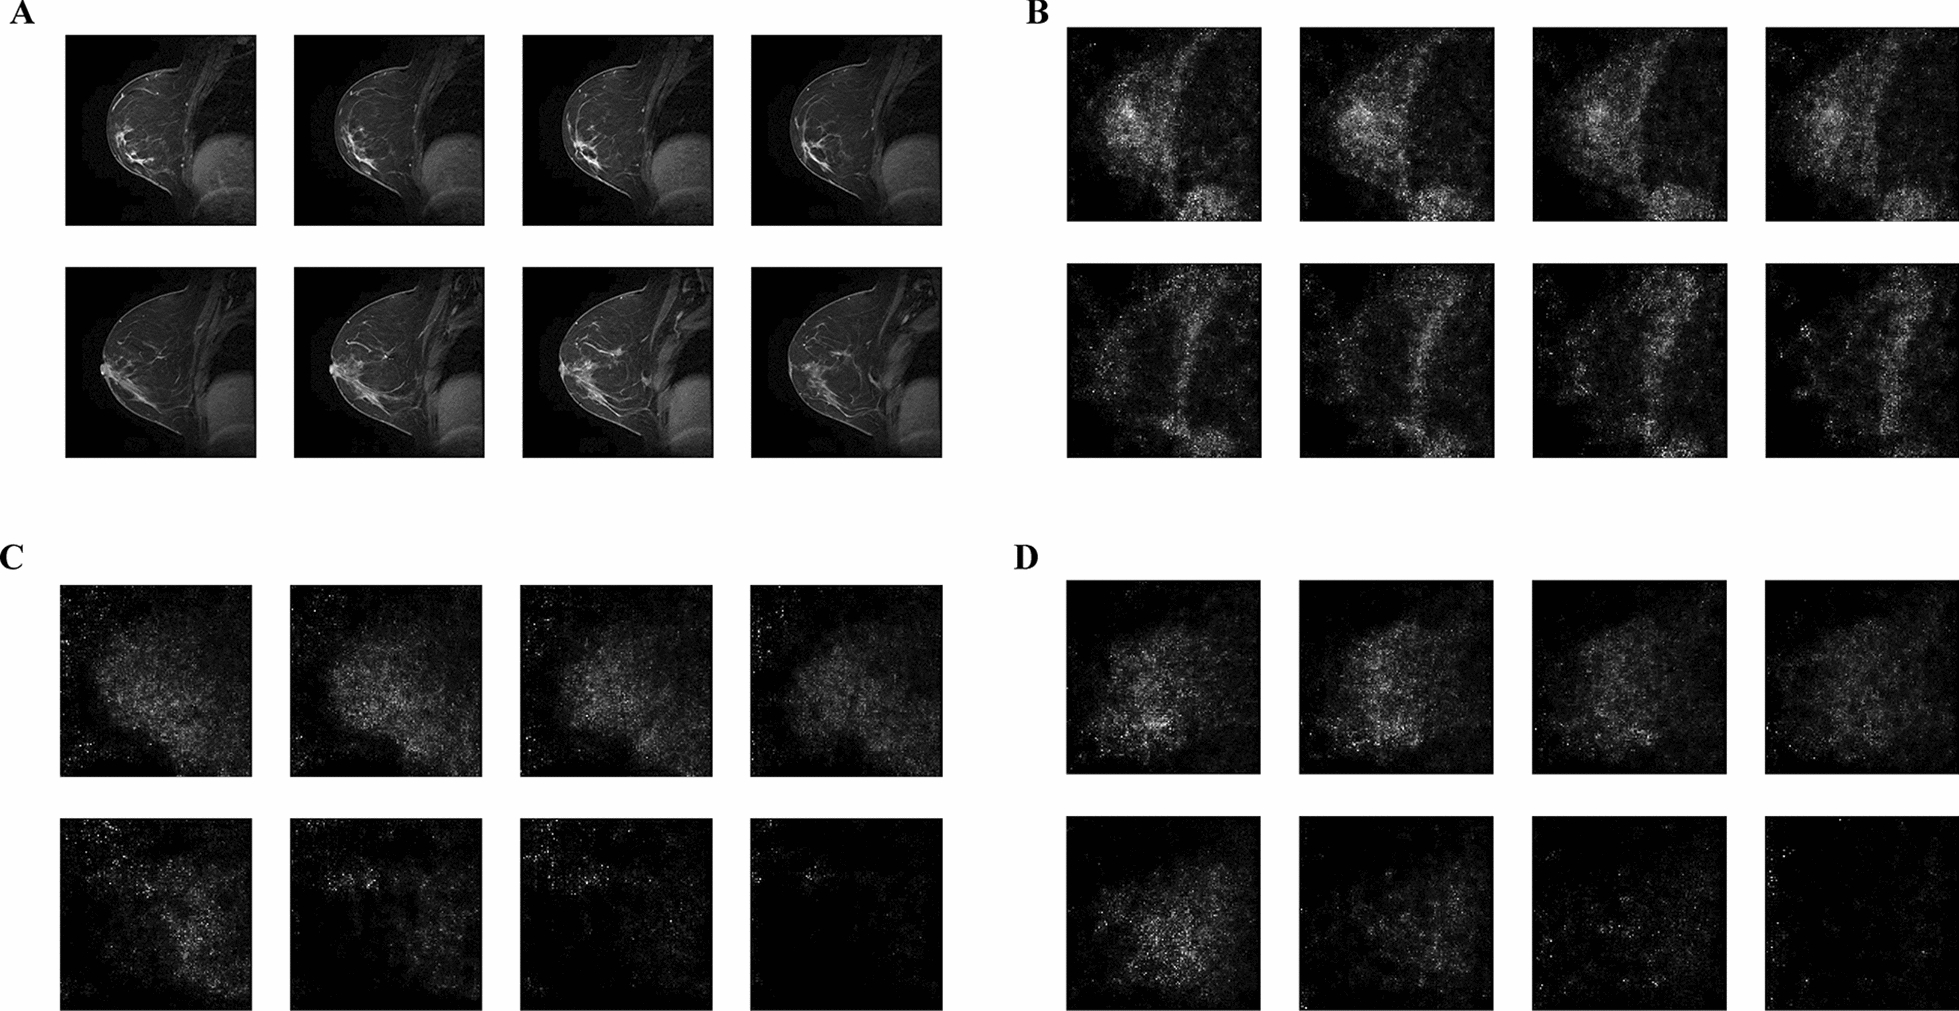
8 slices of 32 total slices of the 3D MRI BC images of patient TCGA-AO-A12E. A Original patient MRI visualized in 32 slices. B Synthetically generated MRI from well-trained cGAN. C Synthetically generated MRI from well-trained autoencoder with Resnet 18. D Synthetically generated MRI from well-trained autoencoder
